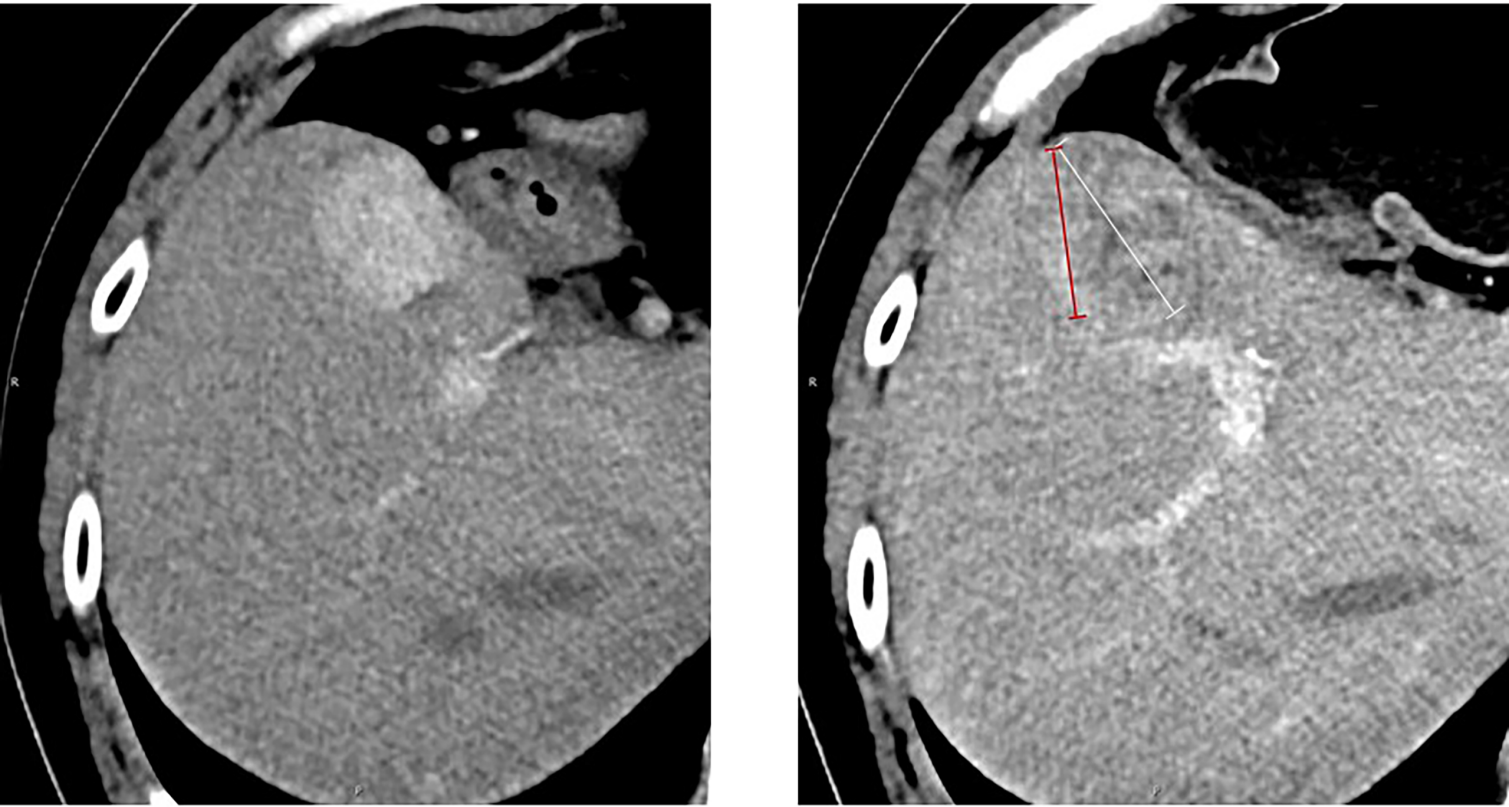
Measurement of the longest tumor diameter in a target hepatic lesion: mRECIST vs. RECIST 1.1. Arterial-phase CT scan obtained after immunotherapy. According to RECIST 1.1, the overall longest diameter of the tumor is captured (white arrow), regardless of the presence of a large area of intratumoral treatment-induced necrosis. In contrast, mRECIST measurement (red arrow) only includes the longest diameter of the viable portion of the tumor, as recognized by contrast enhancement. A 54‐year‐old man who had an arterial-phase CT examination before and after 27 cycles of immunotherapy. The baseline CT size of the target hepatic lesion was 30 mm (left). After immunotherapy, follow‐up CT (right) showed treatment response of previous lesions. The response was assessed as PD according to RECIST 1.1 (47 mm) and SD based on mRECIST (34 mm).
